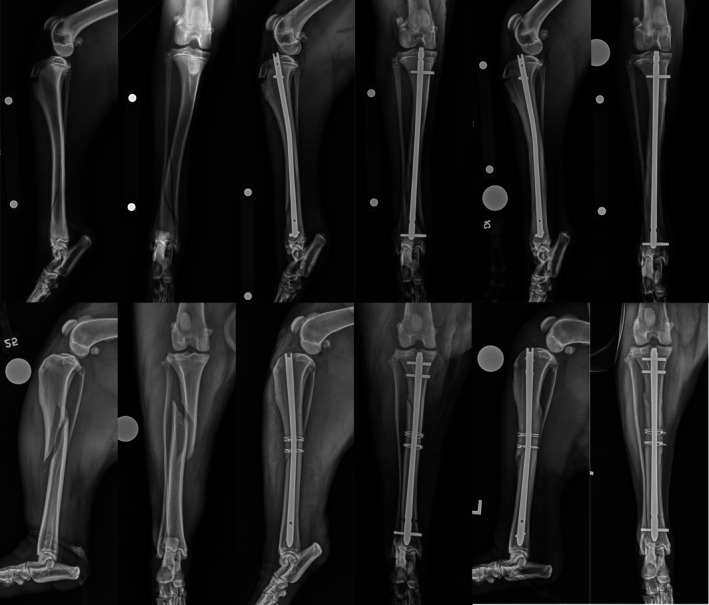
Bolt configurations. Top row – Preoperative, immediate and 3‐week postoperative radiographs of a 5‐month‐old German Shorthaired Pointer with a spiral fracture of the distal tibial diaphysis. The fracture was stabilized using a 6 × 185 I‐Loc AS‐ILN in 1:1 configuration via minimally invasive nail osteosynthesis (MINO). Bottom row – Preoperative, immediate and 6‐week postoperative radiographs of a 2‐year‐old Labrador Retriever with a long oblique fracture of the mid‐to‐proximal tibial diaphysis. The fracture was stabilized using a 7 × 185 mm I‐Loc AS‐ILN in 2:1 configuration via open reduction and internal fixation (ORIF) with two double‐loop cerclage wires.
